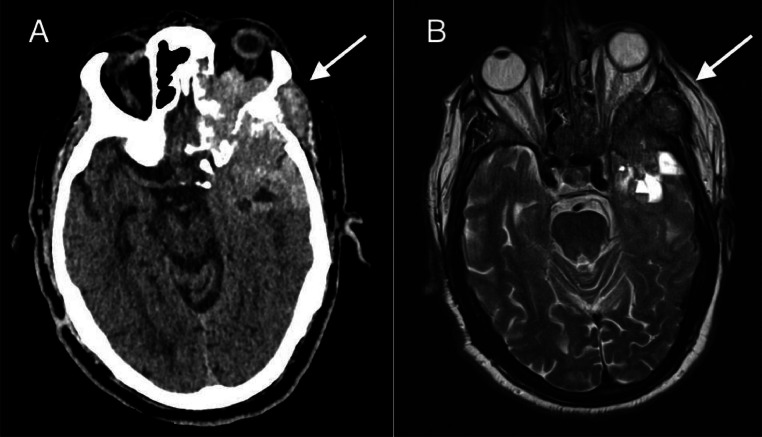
(A) Axial contrast-enhanced CT of the brain showed an irregular infiltrative hyperdense mass in the left temporal region (arrow), characterized by necrotic components and extensive osseous destruction. The mass exhibited orbital, sinusal and muscular extension. Exophthalmos on the left side, adjacent vasogenic edema with increased mass effect and some midline-shift were observed. (B) T2-weighted MRI image showed an hyperintense lesion left temporal with osseous extension (arrow).
